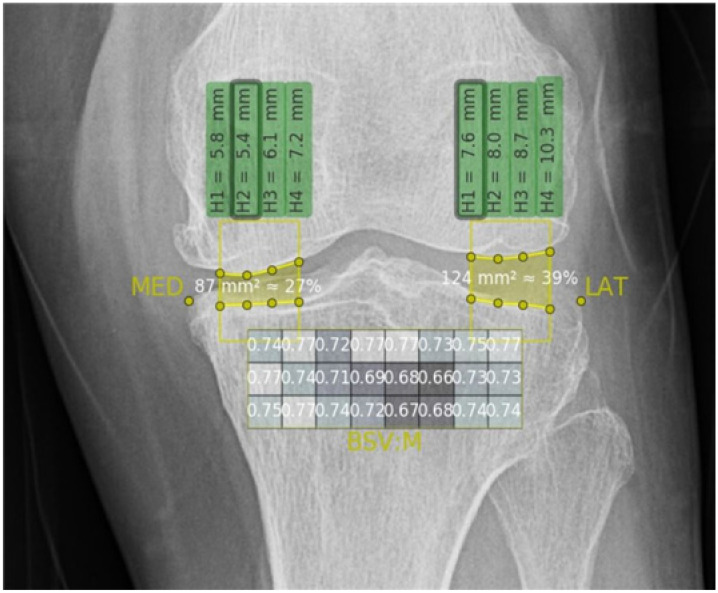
The 24 compartments divided by artificial intelligence software. The artificial intelligence software divided the trabecular bone into 24 compartments at the proximal diaphysis of the tibia in a knee X-ray, showing the BSV results of each compartment. BSV, bone structure value.
